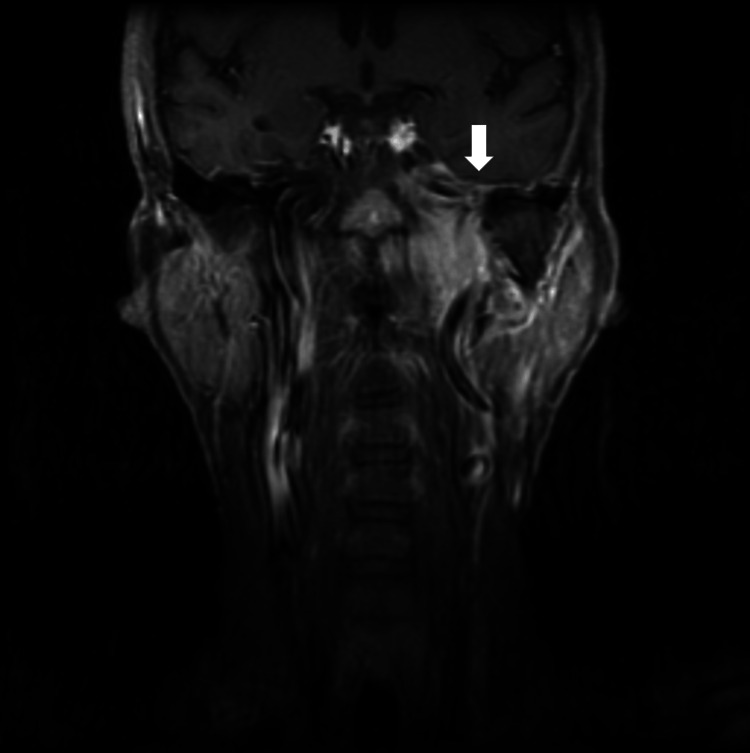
Post-contrast T1-weighted coronal MR image of nasopharyngeal carcinoma in a 59-year-old male patient The case was initially reported as T3; however, a final classification of T4 was made based on the LLM flag indicating cranial nerve involvement. The arrow indicates the area of cranial nerve involvement. LLM: large language model
